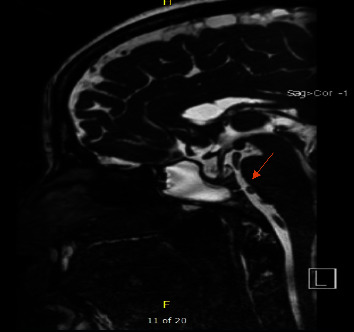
MRI skull base with IV contrast sagittal cut showing fluid accumulation in sphenoid communicating with clival defect (see arrow).
